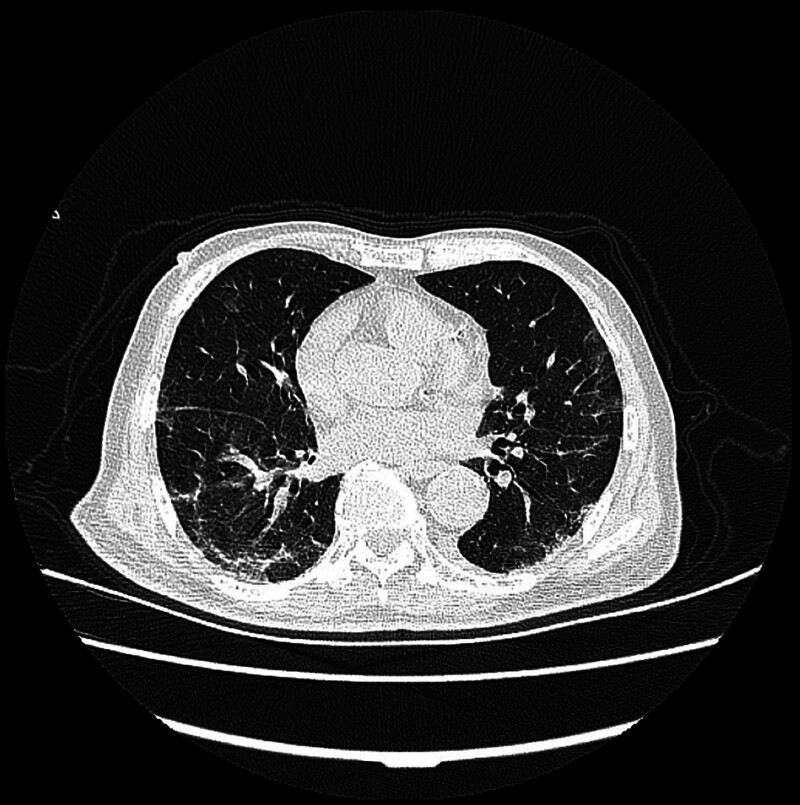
Chest CT image after treatment.
